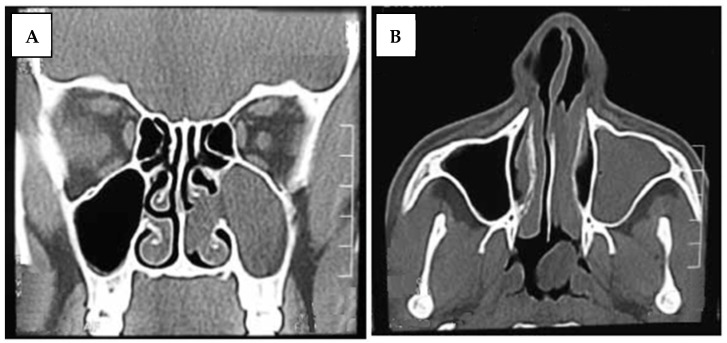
Coronal and axial CT image of an antrochoanal polyp on the left side with extension of the polyp to the nasopharynx (A): coronal and (B): axial CT image.
